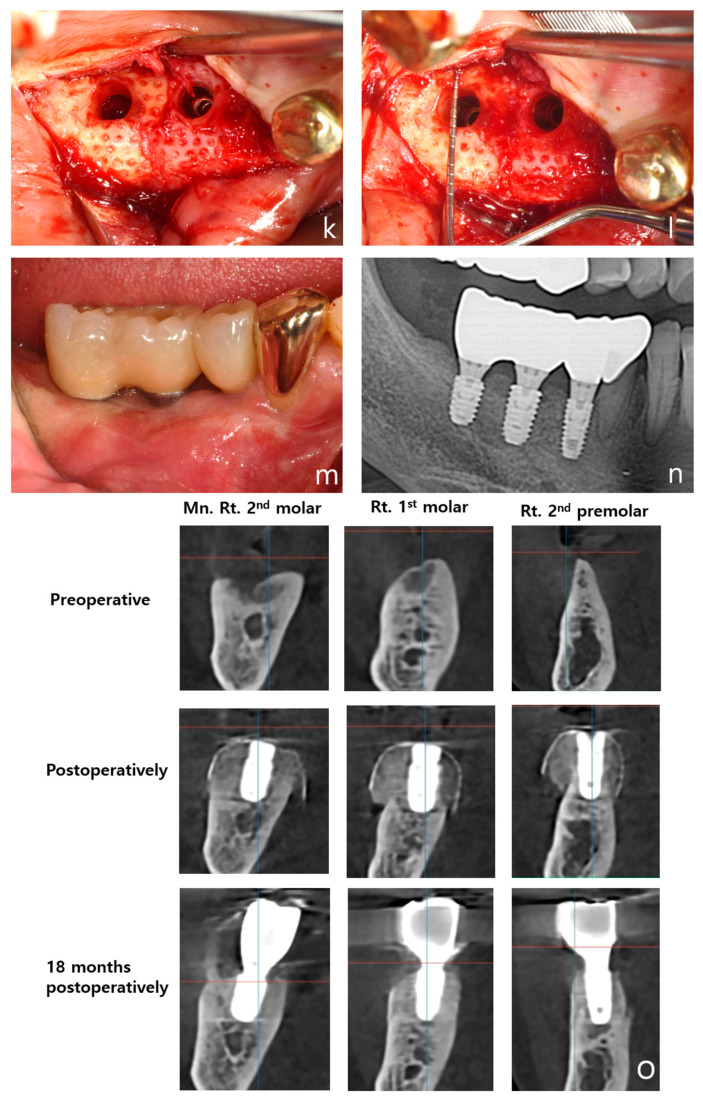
Case 3: Vertical and horizontal bone augmentation with implant placement in the mandibular posterior region of a patient with a history of MRONJ. (a) Pre-operative panoramic radiograph. (b) Post-operative panoramic radiograph. (c,d) Pre-operative intraoral photograph of the surgical site. (e) Severe vertical alveolar bone deficiency was observed after flap reflection following the incision at the surgical site. (f) Approximately 5 mm of thread exposure was observed on the fixture of the first molar (5.0 × 8.5 mm) and the second molar (5.0 × 7 mm). (g) After securing a 3 mm anchor, allograft and xenograft were layered for bone augmentation. (h) Application of vertical-type Ossbuilder Ti-mesh and securing of the cover cap. (i,j) Intraoral view at 4 months post-surgery. (k,l) High-quality alveolar bone formation was noted around the fixtures during the second surgery. (m) Intraoral view at 18 months post-operation. (n) Panoramic radiograph after placement of the final prosthesis. (o) Pre-operative, post-operative, and 18-month post-operative CT images (sagittal view) for each implant site. The vertically augmented bone graft material is observed to be well-maintained and remodeled.
